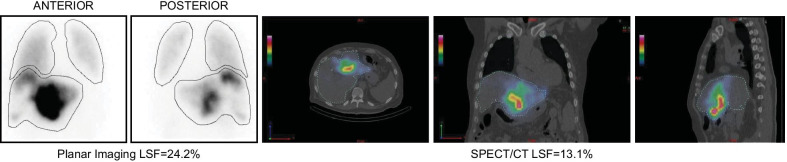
Significantly higher LSF of 24.2% from ^99m^Tc-MAA planar imaging versus LSF of 13.1% from ^99m^Tc-MAA SPECT/CT (patient 43; Table 3)
